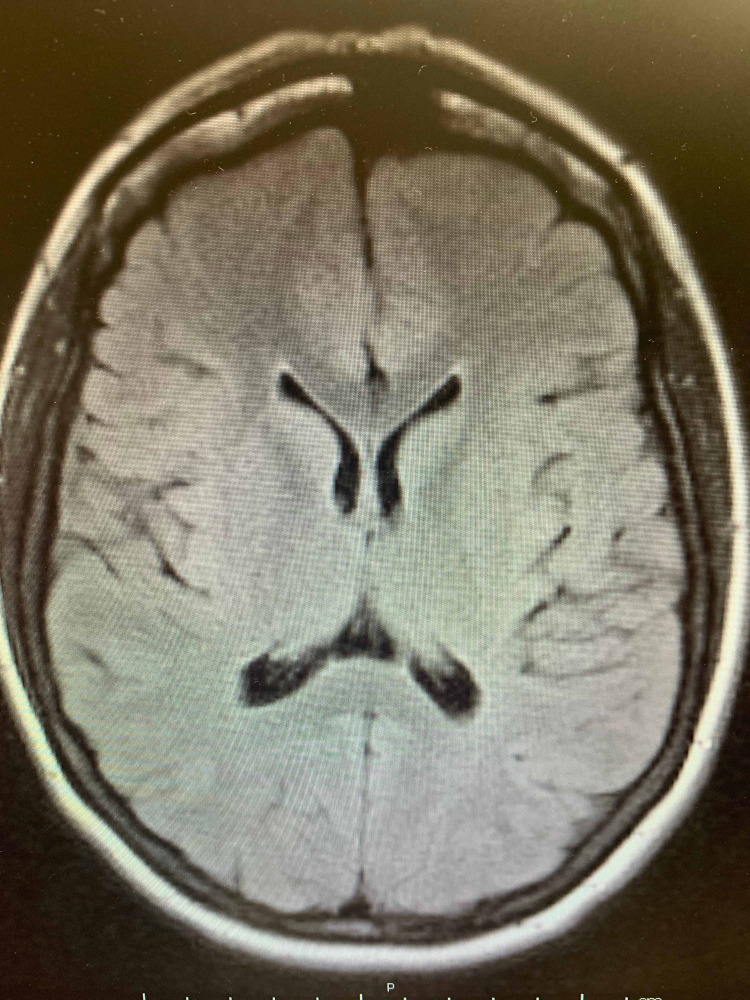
FLAIR MRI: normal brain. FLAIR-MRI brain: fluid-attenuated inversion recovery magnetic resonance imaging of the brain
